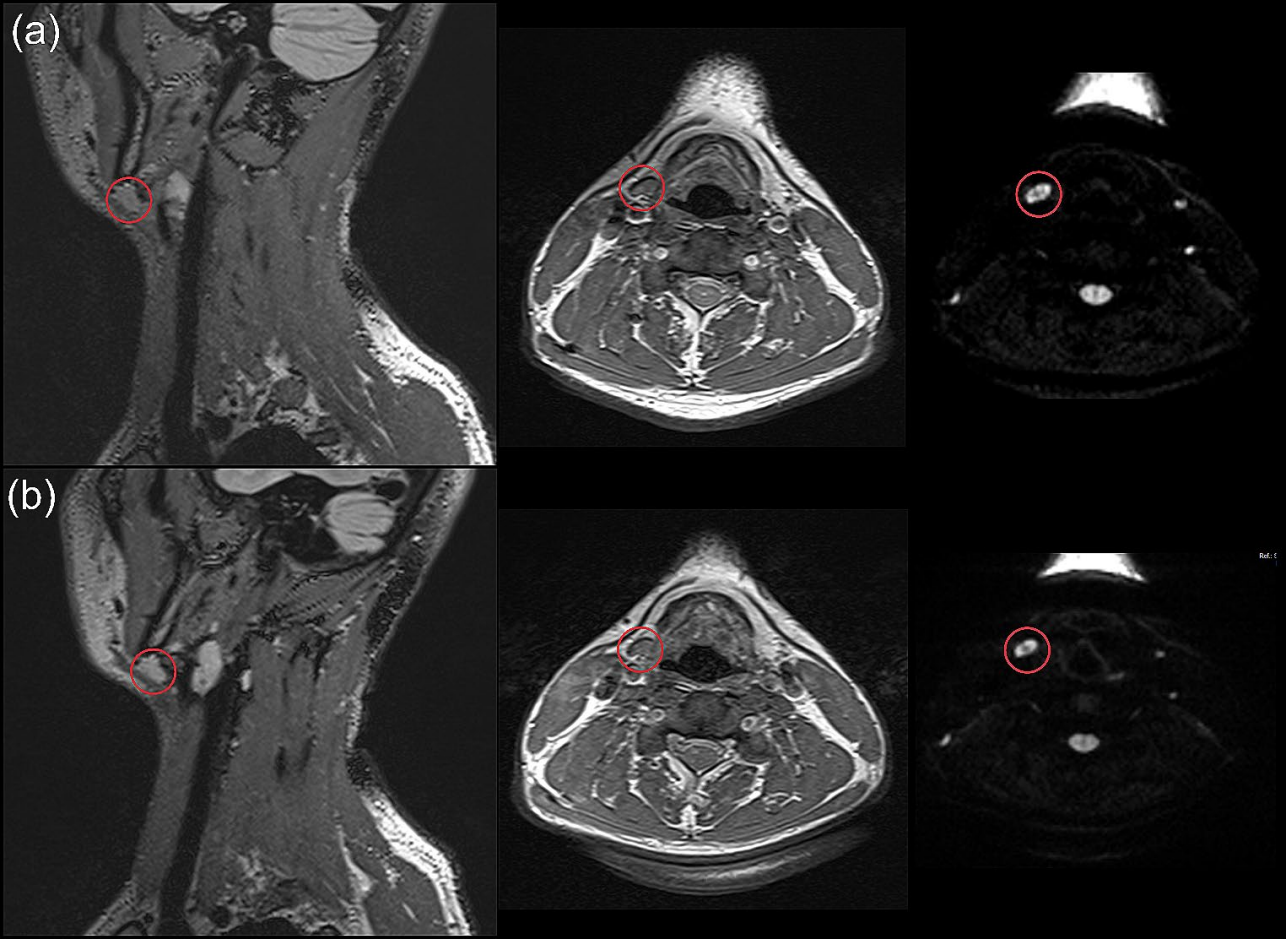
Exemplary MRI data of a living strangulation victim with no findings in digital photography, but a hematoma in the infrared photography (see also Fig. 3). In the MRI data, a hemorrhage of the cervical lymph node was detected in both MRI scans (red circle). (a) MRI scan acquired within timeframe 1 (3 days) after the incident, (b) MRI scan acquired within timeframe 2 (9 days) after the incident. Left: 3D FLAIR sequence (sagittal view); Middle: T_1_-weighted TSE DIXON in-phase data (transversal view); Right: trace-weighted RESOLVE diffusion sequence (transversal view)
